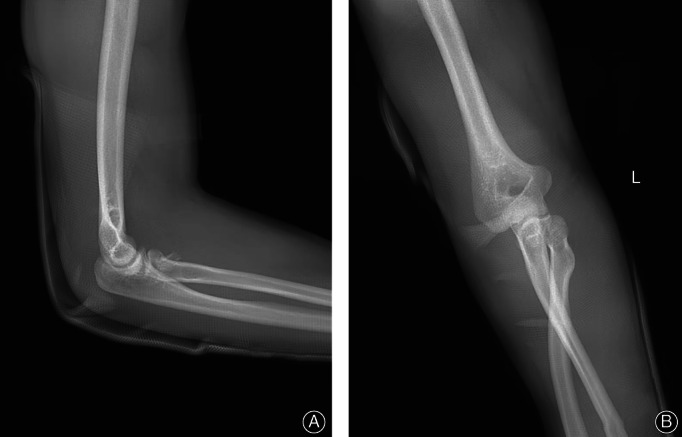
(A) Lateral view of the elbow after reduction showing that the ulnar joint returned to normal. (B) Anteroposterior radiographs after reduction showed recovery of the ulnar joint, asymmetry of the brachioradial joint, and displacement of the proximal radius to the medial side of the ulna
